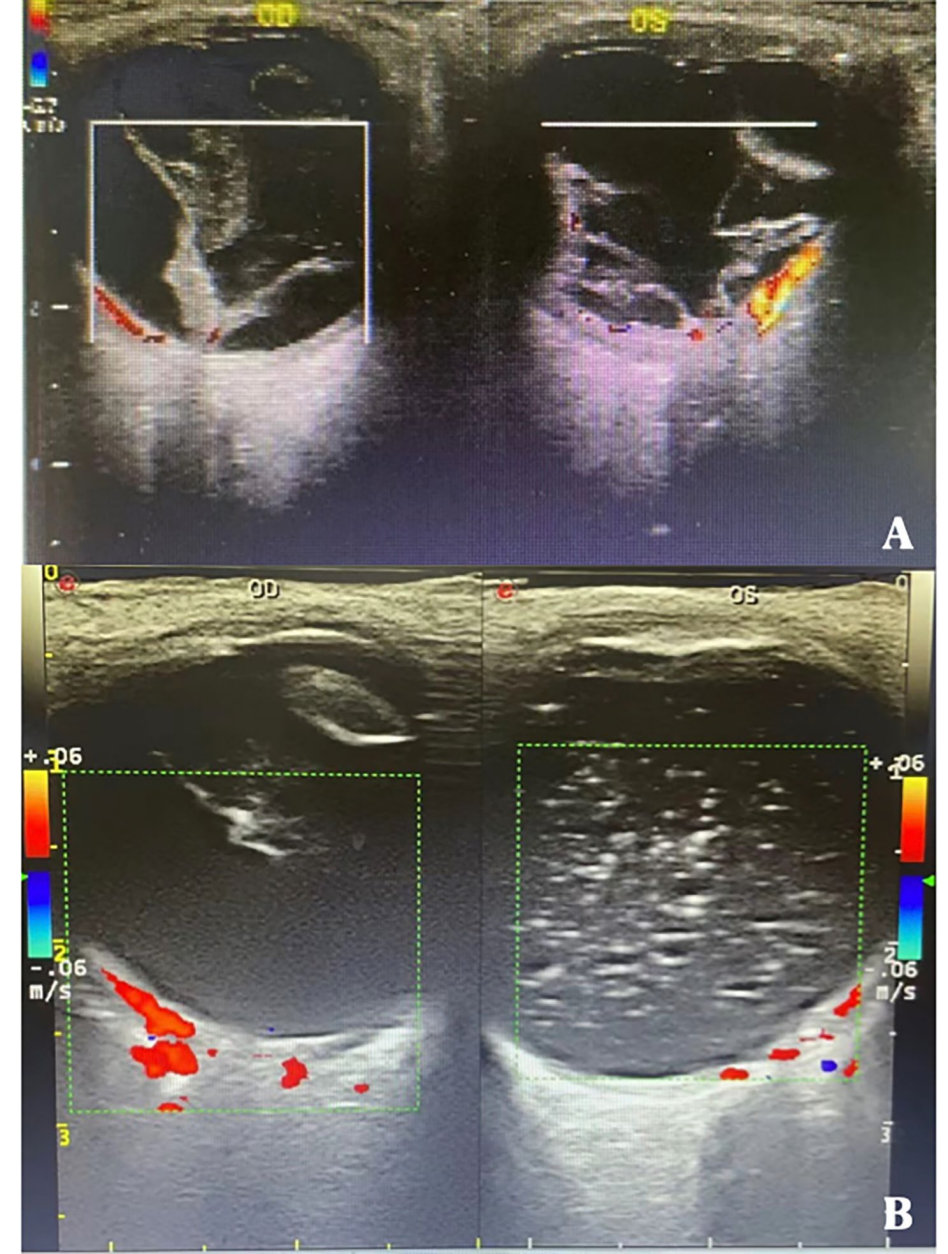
Ocular ultrasound of the patient. (A) During the patient’s initial visit to our hospital, binocular ultrasonography showed vitreous opacity and retinal detachment in both eyes. (B) Four months after the removal of the silicone oil in the left eye, ocular ultrasonography showed residual silicone oil in the left eye, and the retina was in place, while the retinal detachment in the right eye was spontaneously reattached without any surgery
